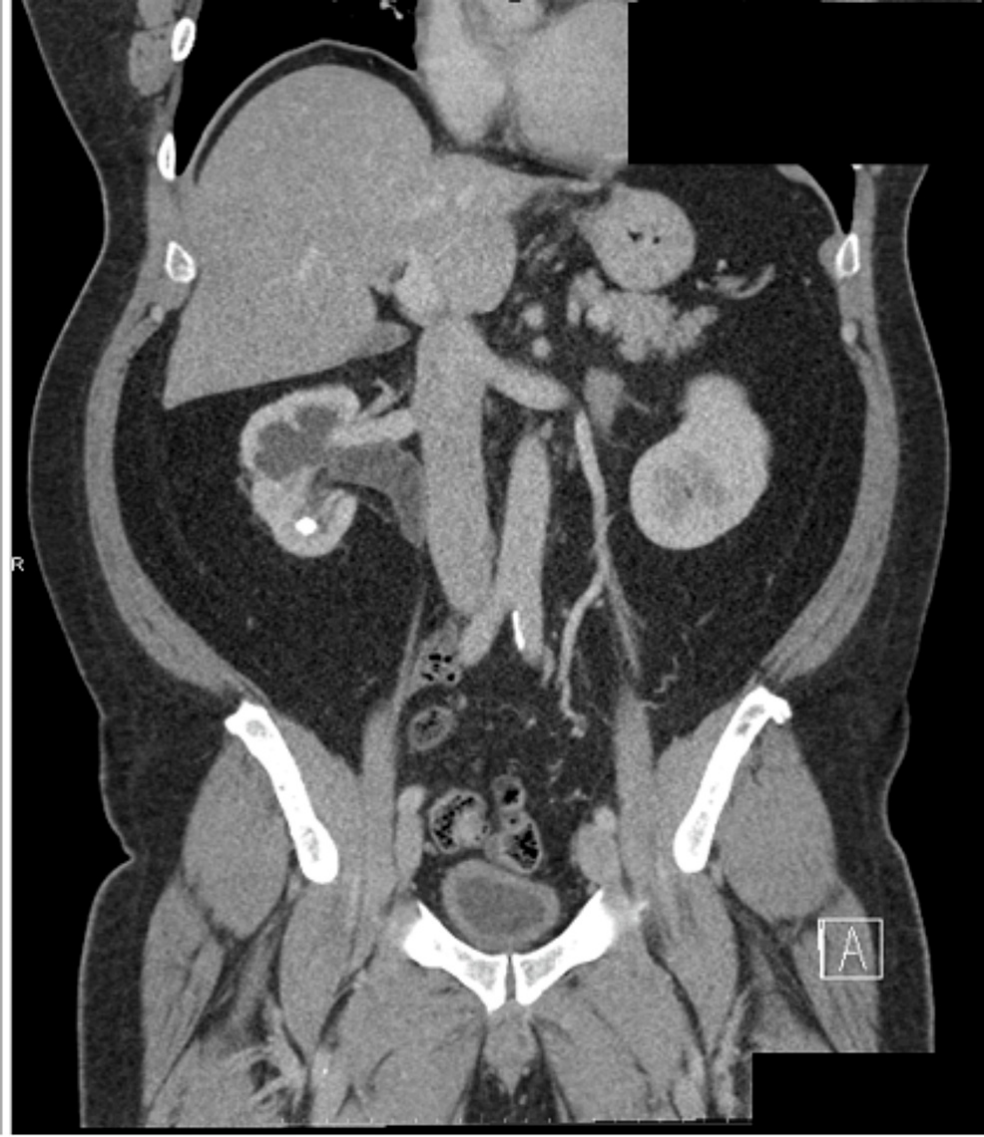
Coronal view of computed tomography of the abdomen/pelvis with intravenous contrast. Retrocaval ureter with incidental nephrolithiasis.
